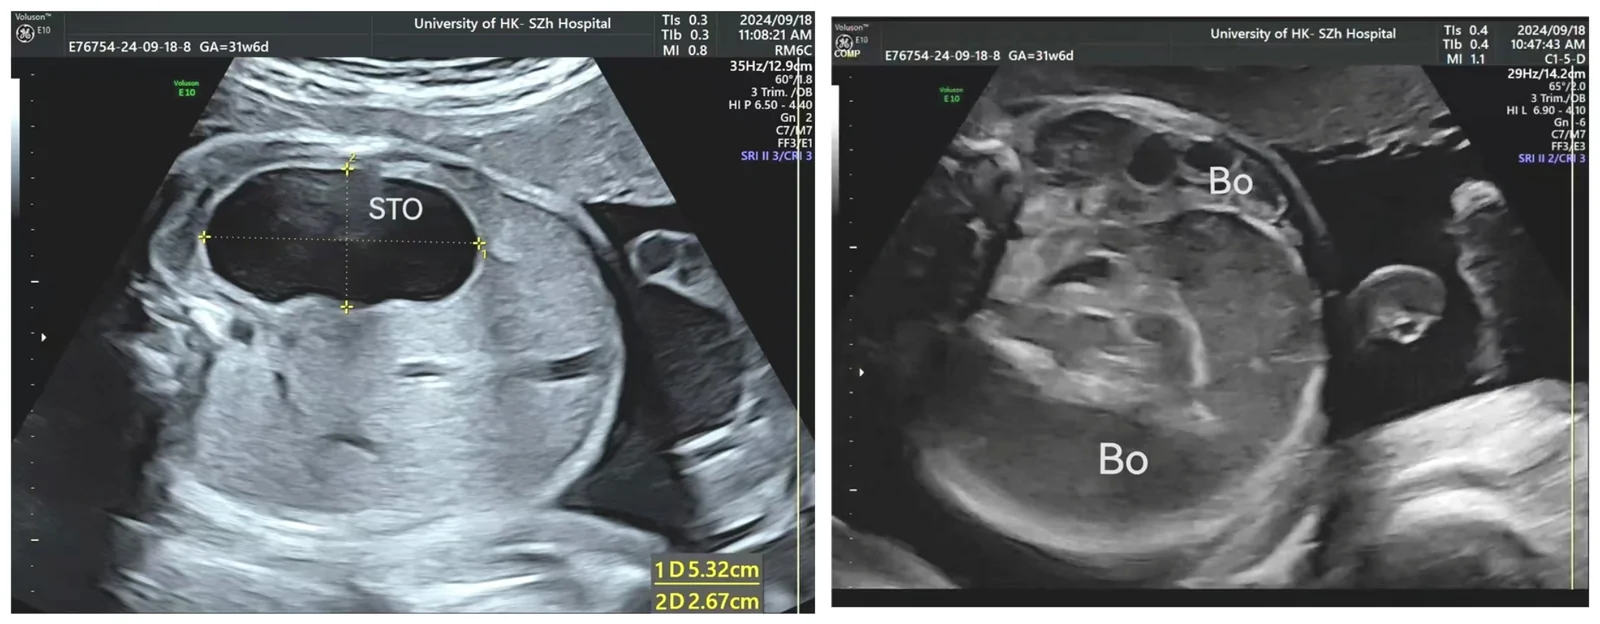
Fetal ultrasound (31 weeks): A large fetal stomach (left image) and a dilated fetal bowel (right image). STO: stomach; Bo: bowel. 1: anteroposterior diameter, 2: transverse diameter.
